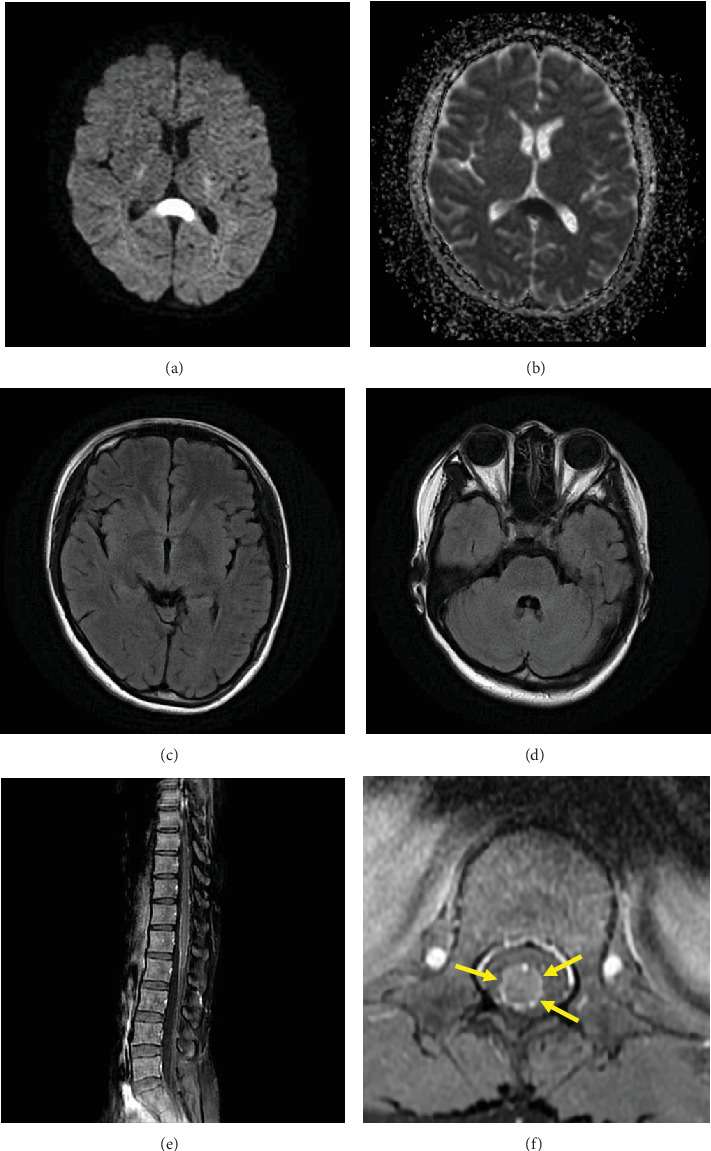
Magnetic resonance imaging (MRI) at hospitalization. Diffusion-weighted imaging (DWI) showed high signal intensity in the splenium of the corpus callosum (a). Signal reduction in the same area was observed on the ADC map (b). FLAIR images did not show any abnormal signal areas other than the splenium of the corpus callosum (c, d). Sagittal and axial T1-weighted contrast-enhanced images of the lower spine (e, f) showed contrast enhancement on the surface of conus medullaris (f, arrows) and expansion of the surrounding vessels.
